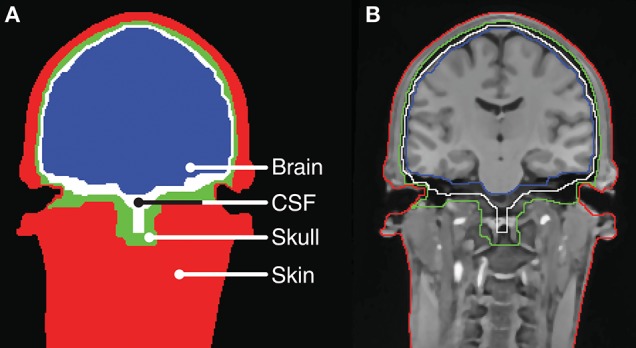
Tissue boundaries for a slice of the head model for Subject C. (A) Tissue segments. (B) MRI scan with indication of the tissue boundaries.
